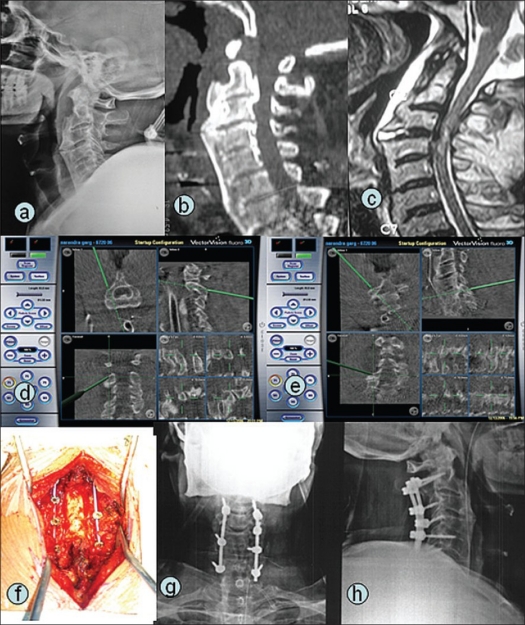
A 58-year-old male (case 12) with ankylosing spondylitis sustained chance fracture at C3-C4 (a,b,c) with ASIA grade B neurological deficit. Posterior instrumented fusion and decompression was planned. Intraoperative navigation pictures (d,e) showing multiplanar pedicle entry point and trajectory localization. Intraoperative photograph (f) and postoperative radiographs showing good placement of pedicle and lateral mass screws (g,h)
